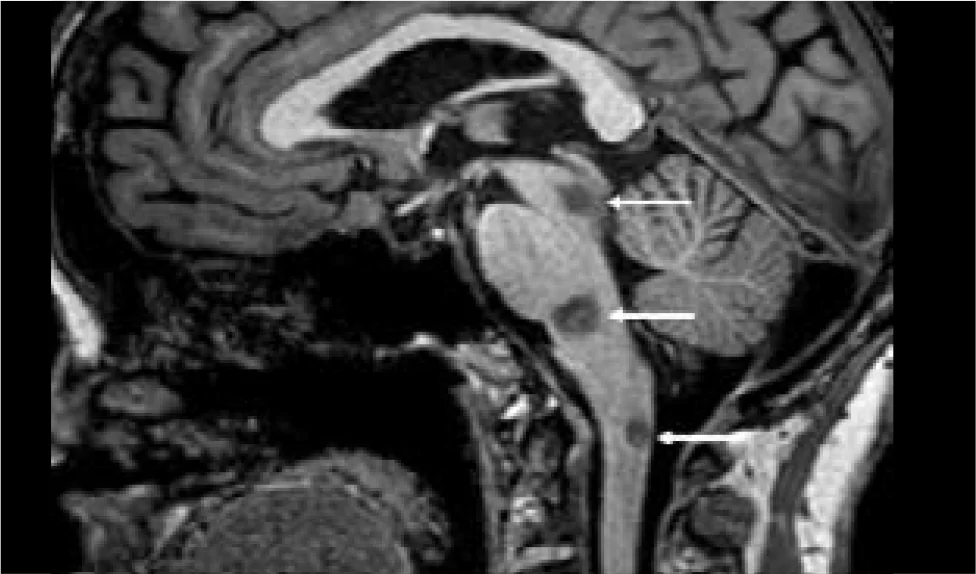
MRI of a 67-year-old woman presenting with left-sided
